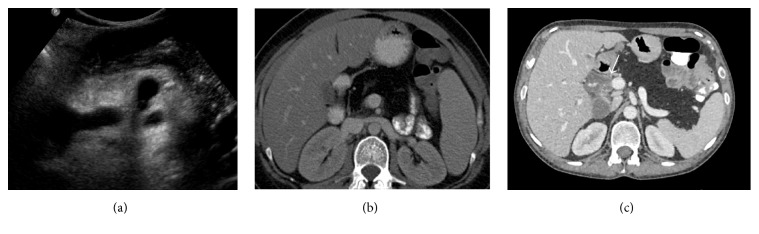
(a) Transverse abdominal ultrasound of a 34-year-old male with cystic fibrosis. Fatty infiltration of the pancreas is typically echogenic on US. (b) The pancreas is homogenously low in attenuation on CT and is often difficult to differentiate from normal retroperitoneal fat. (c) Axial portal venous phase contrast-enhanced CT performed to assess abdominal pain in a 44-year-old CF patient 2 weeks after bilateral double lung transplant demonstrates diffuse pancreatic lipomatosis which manifests as homogenous low attenuation. Note is also made of a distended common bile duct (white arrow) due to stricture.
